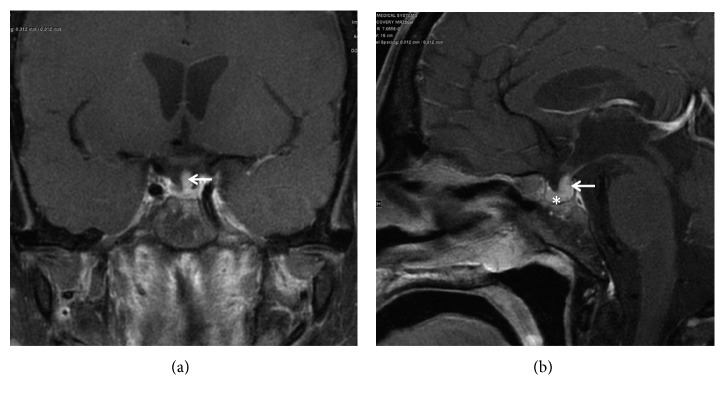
(a) Coronal and (b) sagittal pituitary MRI images post-gadolinium contrast show thickened enhancing pituitary stalk (arrow). It measures 4.7 mm at the level of the optic chiasm. The pituitary gland is within normal limits (asterisk).
